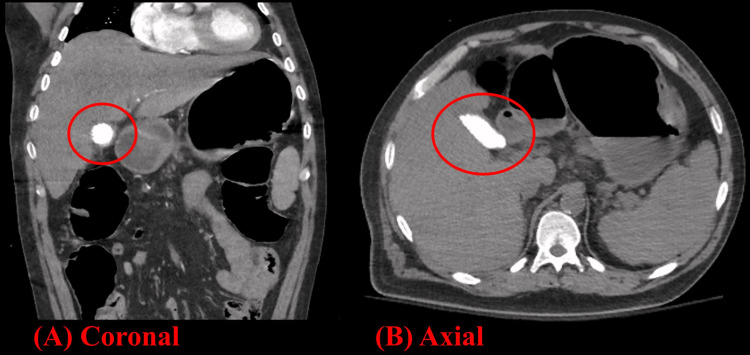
CT abdomen and pelvis coronal (A) and axial (B) images showing a diffusely radiopaque gallbladder (circle) consistent with porcelain gallbladder.
